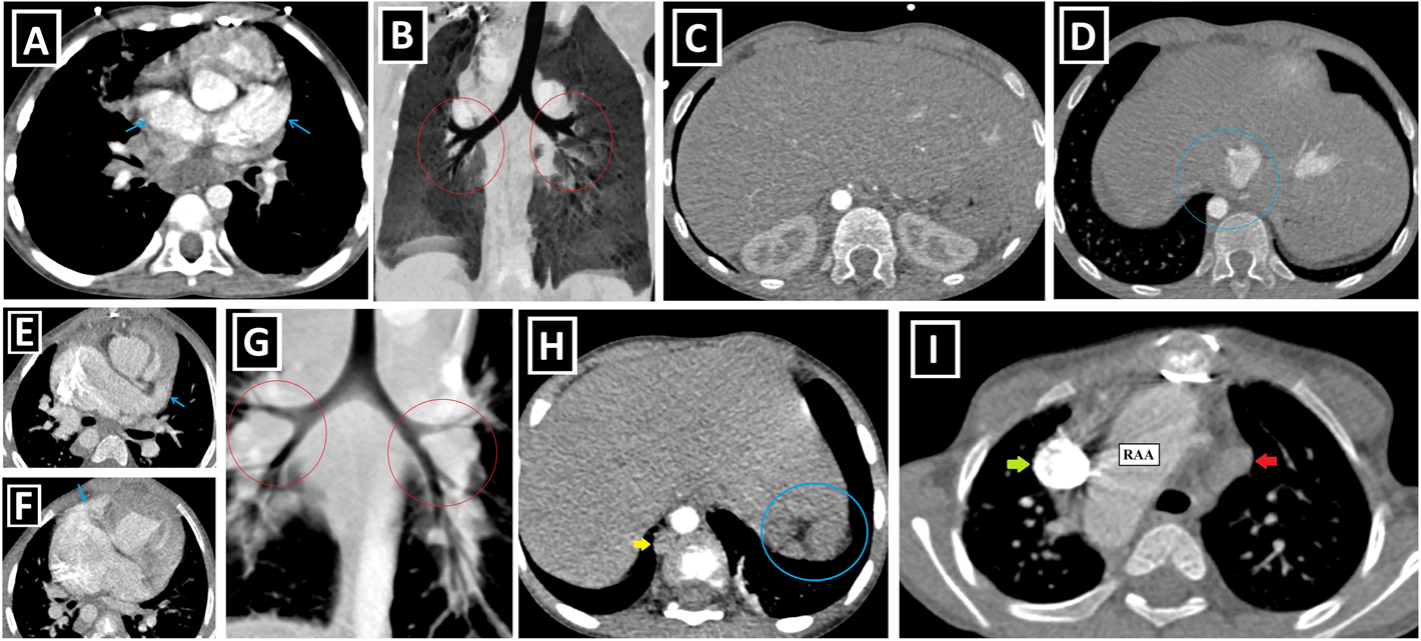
PLSVC with heterotaxy-2 (right and left isomerisms). a–d Axial (a, c, d) and coronal-oblique minimum intensity projection (b) CT images depict right isomerism characterized by a bilateral broad-based triangular atrial appendages (blue arrows) (a), bilateral trilobed lungs (red circles) (b), asplenia (c), and juxtaposition of IVC (blue circle) in a patient with PLSVC (not shown). e–i Axial (e, f, h, i) and coronal-oblique reformatted (g) CT images depict left isomerism characterized by a bilateral narrow-based finger-like atrial appendages (blue arrows) (e, f), bilateral bilobed lungs (red circles) (g), polysplenia (blue circle) (h), dilated azygos vein due to the IVC absence (yellow arrow) (h), and right aortic arch (RAA) in a patient with double SVC (green and red arrows) (i)
